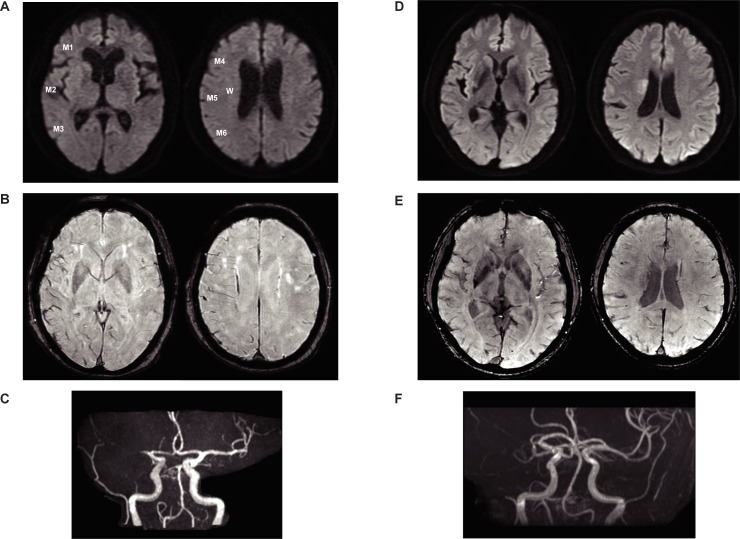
Representative case. Case 1, cardioembolic stroke. (A) Initial diffusion-weighted imaging (DWI)-based modified Alberta Stroke Program Early CT Score (mASPECTS) was 6 points. (B) Initial T2*-weighted MR angiography (SWAN)-based mASPECTS was 0 points. (C) Magnetic resonance angiography (MRA) shows right M1 occlusion. (D) Case 2, atherothrombotic stroke. (E) Initial DWI-based mASPECTS was 6 points. (E) Initial SWAN-based mASPECTS was 5 points. (F) MRA shows right M1 occlusion.
